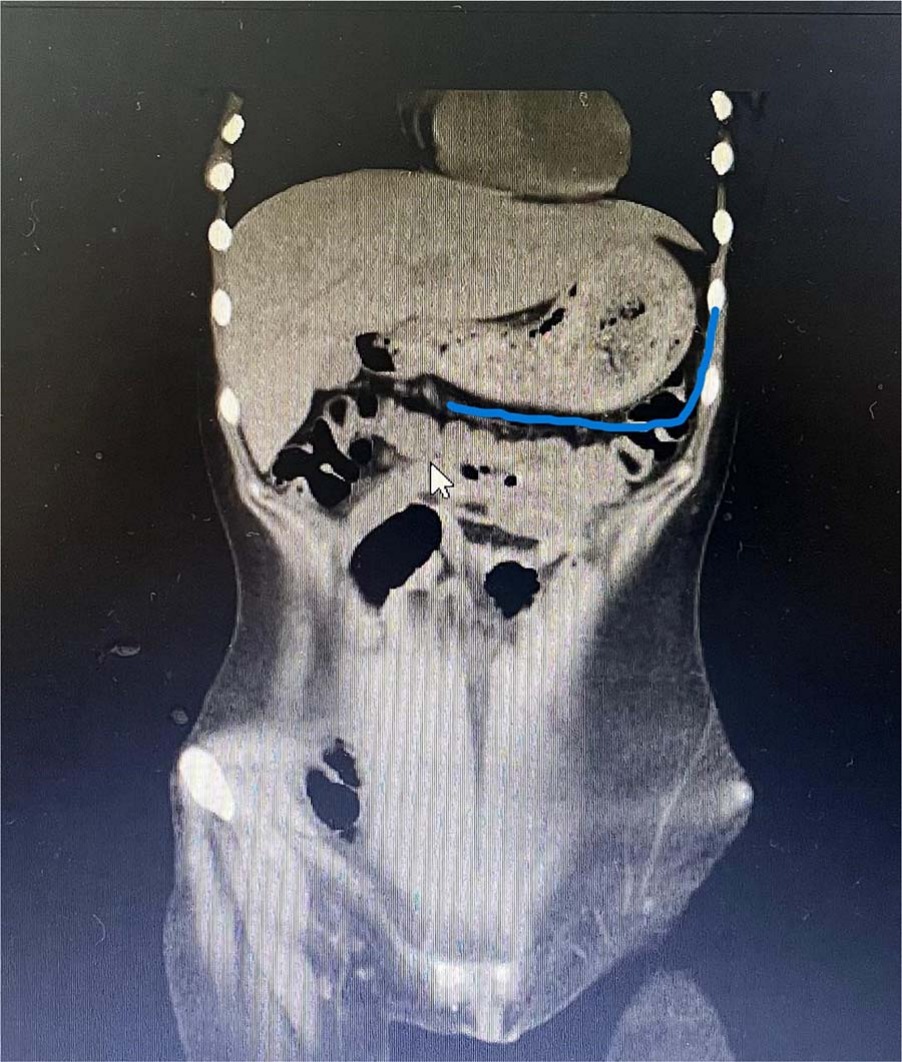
Sagittal CT image demonstrating the foreign body filling the entire gastric cavity.
